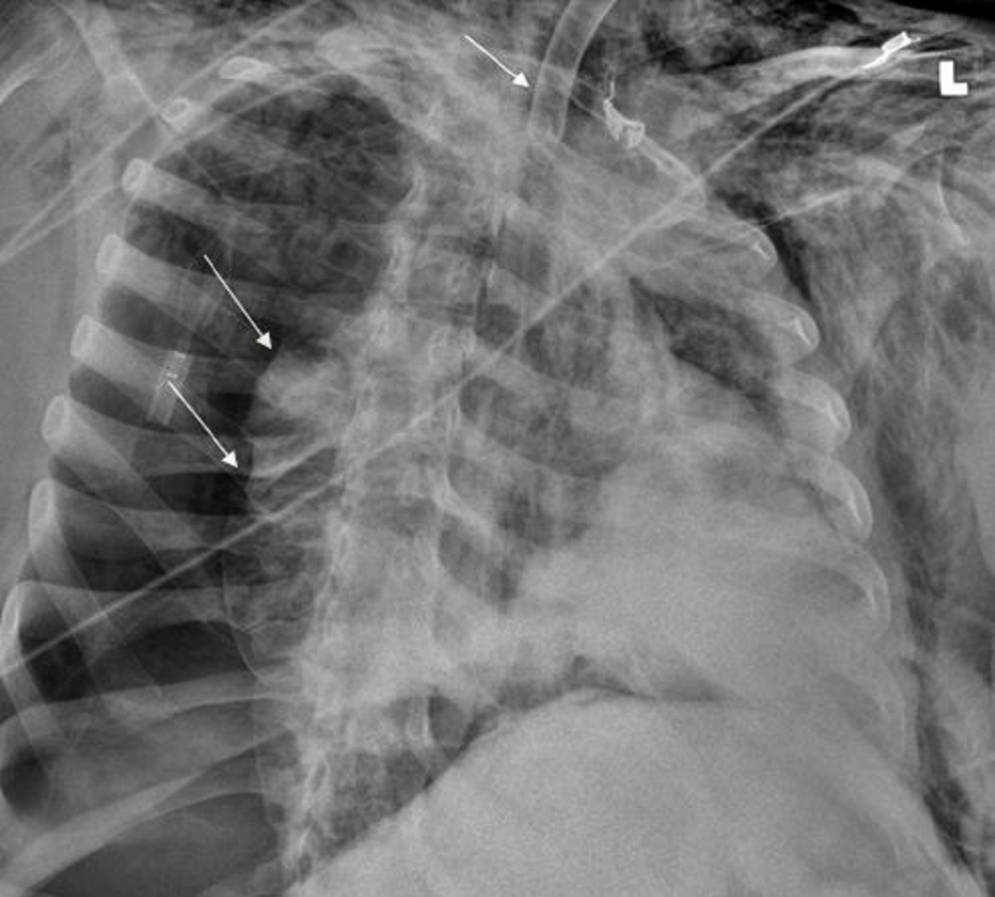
Right-sided pneumothorax as shown by the arrows (Right) on initial chest X-ray with tracheostomy tube repositioned (Top arrow)
